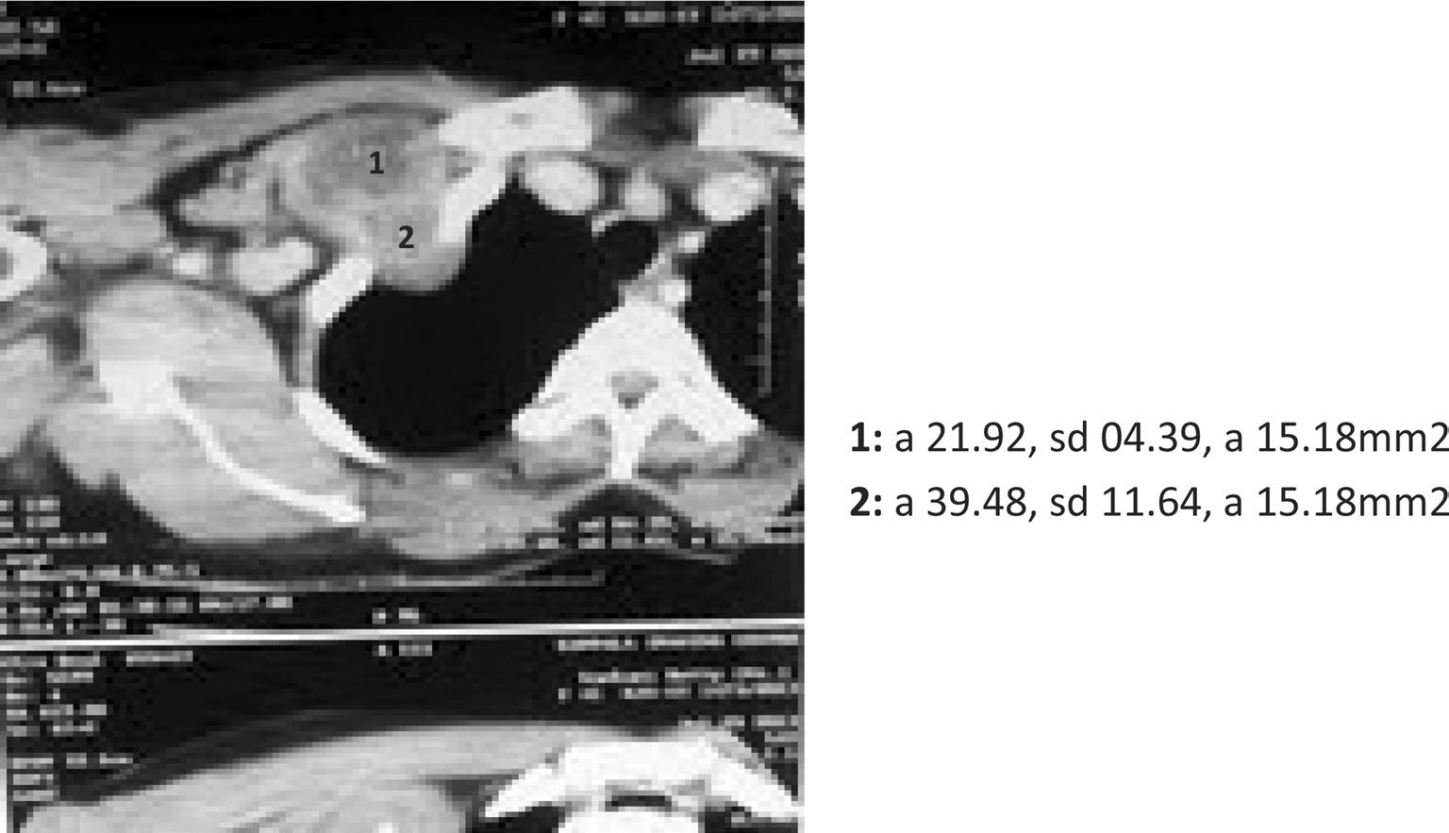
A CT Scan film of a patient with second intercostal nerve schwannoma
